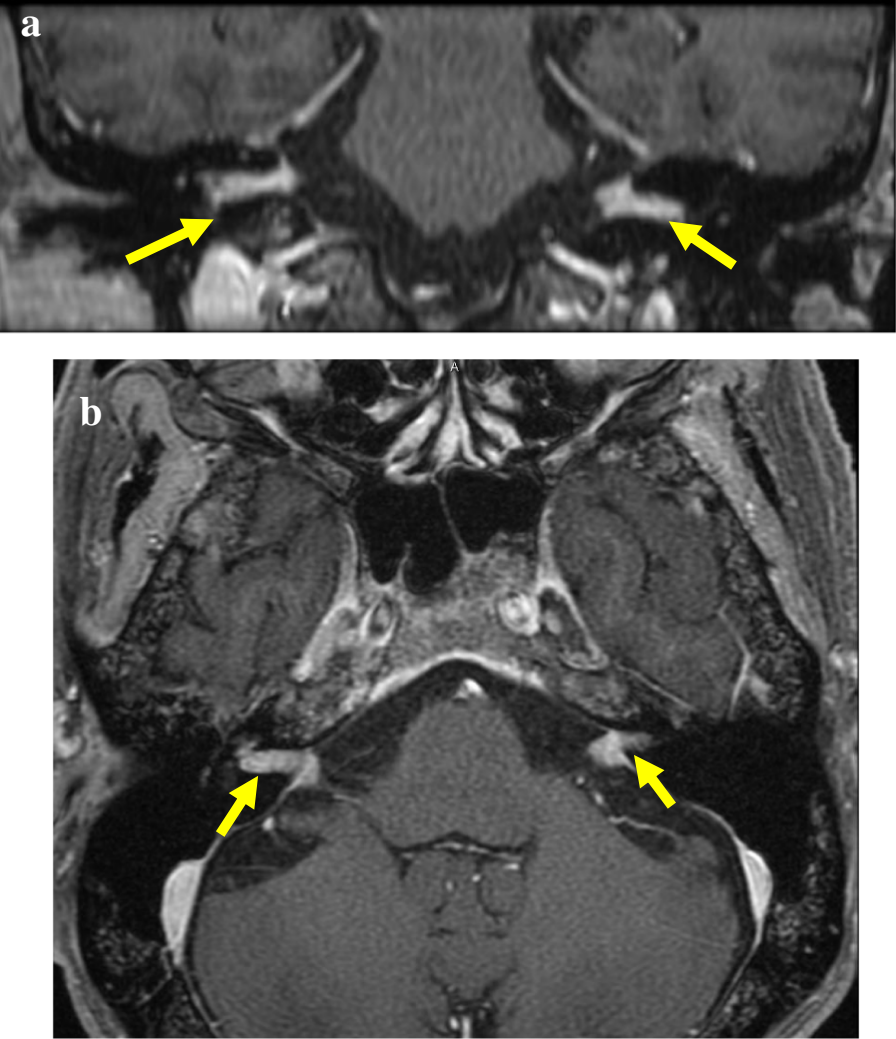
Enhanced MRI scan. a (coronal), b (horizontal): MRI scan showing the neoplastic lesion filling the bilateral internal auditory canal
